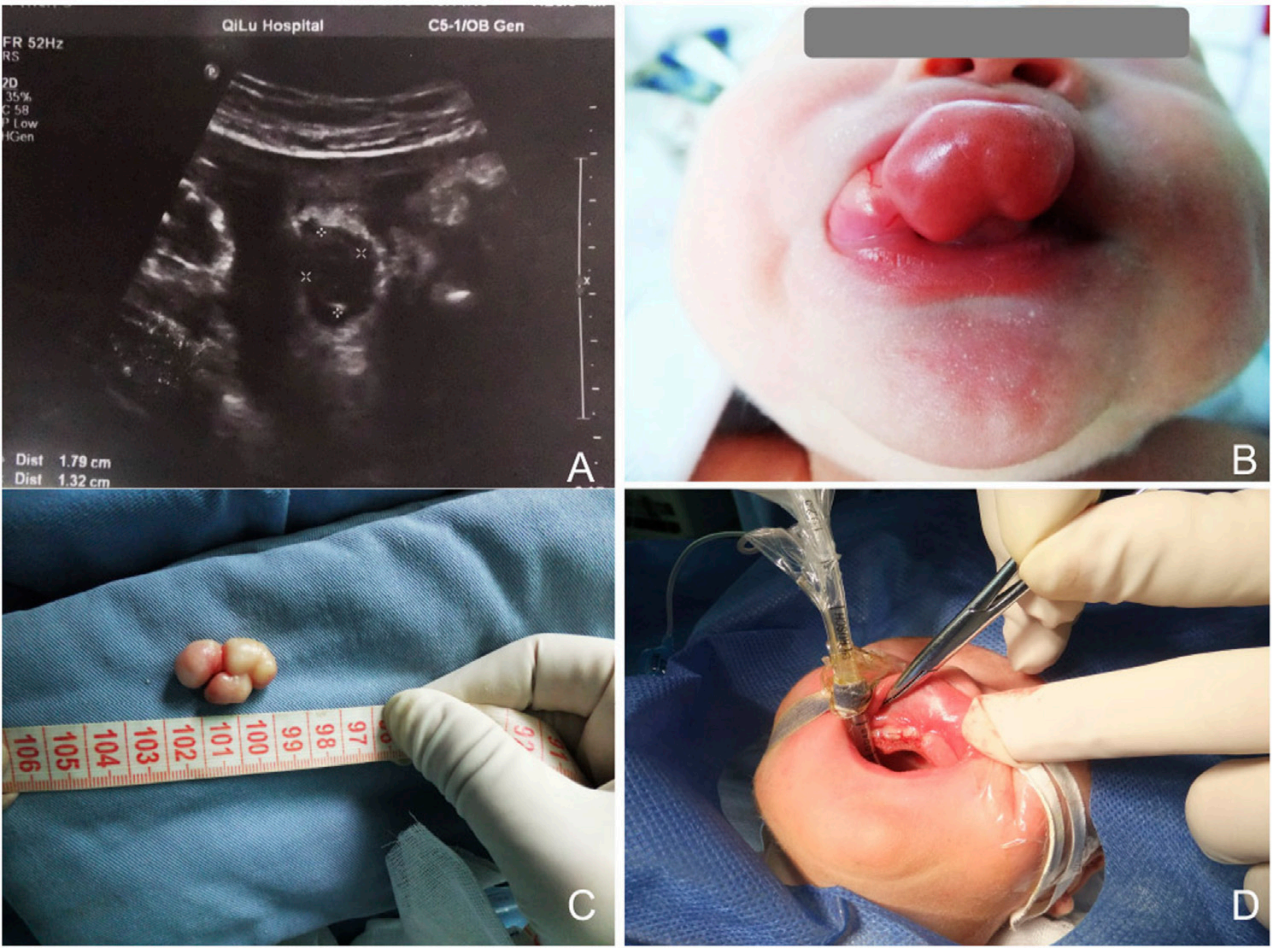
(A) Sonography at 34.5 weeks of gestation, showing a mass protruding from the mouth of the fetus. (B) Appearance of the baby with the oral mass. The lesion was sessile and lobular, with a smooth surface. (C) Gross view of the lesion. (D) Three absorbable sutures were used to close the wound.
